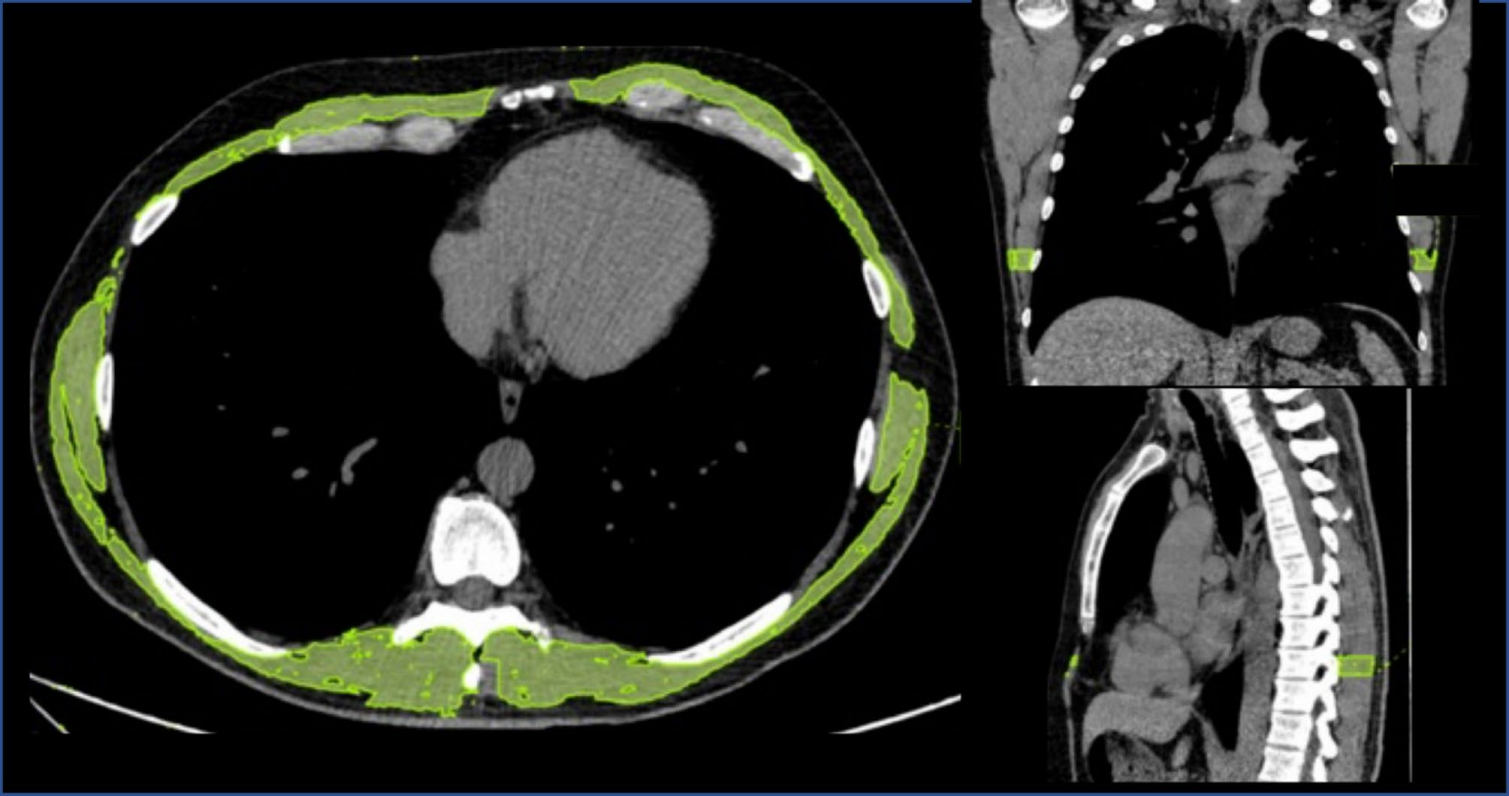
The chest wall muscle area was semi-automatically segmented on axial CT images at the level of the ninth thoracic vertebra, using the mediastinal viewing window. All soft tissues with Hounsfield values comprised between 0 and 100, located outside the rib cage (excluding ribs, vertebrae and lung parenchyma), were segmented (green color) and considered as accessory respiratory muscles (color figure online)
